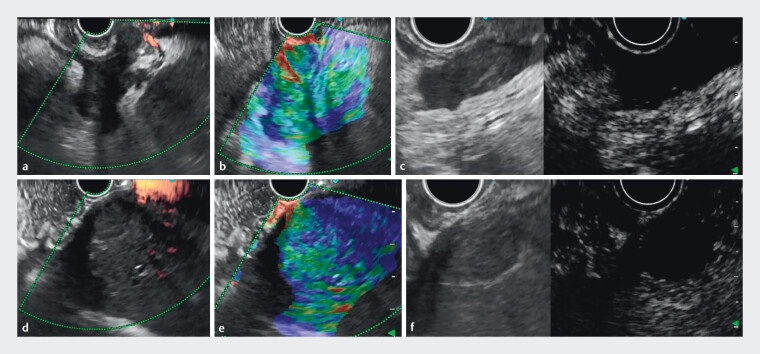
Endoscopic ultrasonography (EUS) revealed multiple hypoechoic masses (1–5 cm) with moderate stiffness in the gastric/duodenal walls, splenic hilum (
